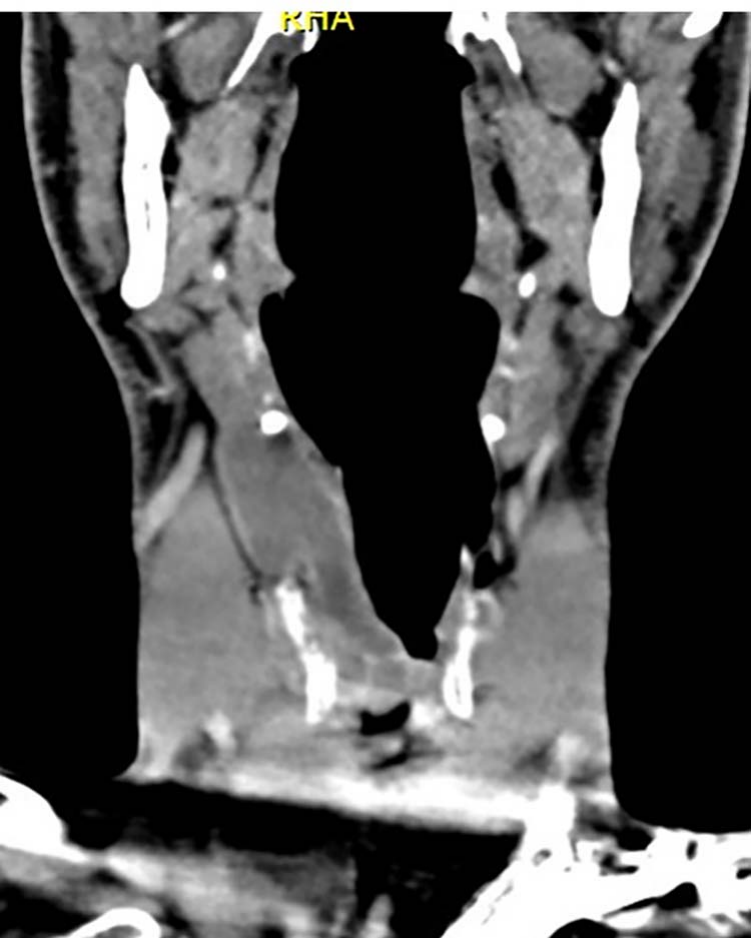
Coronal CT of neck showing the internal and external components of the laryngocoele, in relation to the thyrohyoid membrane.
